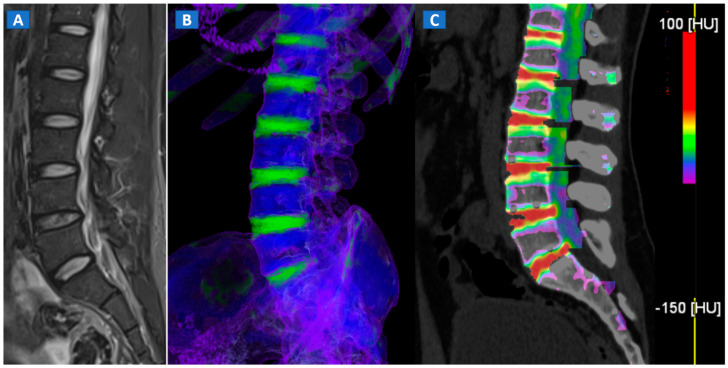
A 43-year-old male with low back pain following high-energy trauma, with no vertebral fractures. On the sagittal STIR MRI image (A), all lumbar vertebral bodies show a normal shape and signal intensity. On the 3D DECT image, on the para-sagittal plane (B), normal vertebral bodies are coded in blue, whereas density increases, due to increased water content, are coded in green. As expected, the normal intervertebral discs are coded in green. In addition, normal cortical bone is also coded in green, due to an incomplete subtraction process. On the corresponding 1 mm sagittal 2D DECT image (C) there is a color lookup table that codes bone marrow and areas of oedema in shades of green-yellow to orange-red. DE-specific information has been fused with conventional grayscale morphological images (thickness, 1 mm; increment, 1 mm). The range of densities is set between −150 and 100 HU, with underlying normal bone visualized without any superimposition for density values below the −50 HU cut-off.
